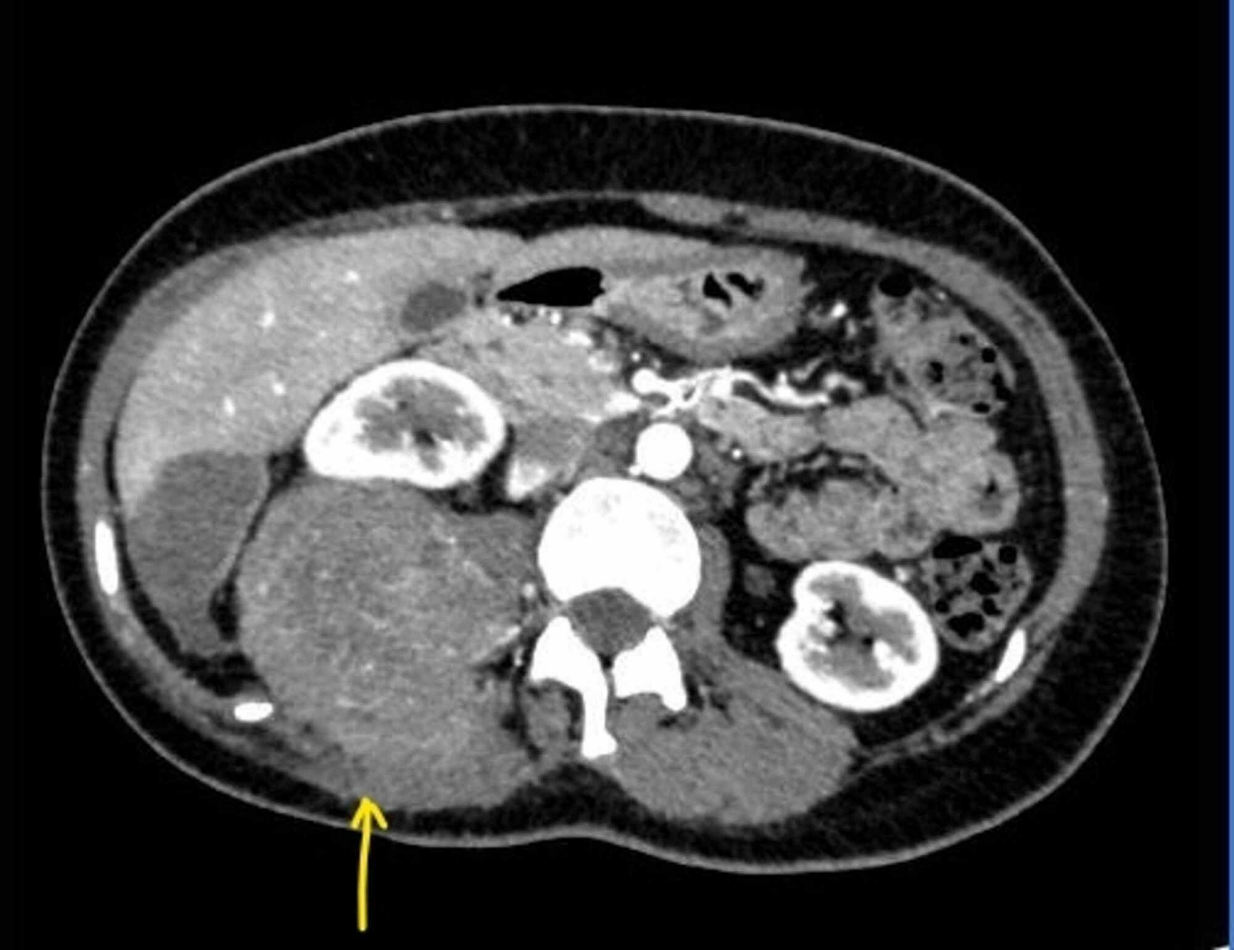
Contrast-enhanced CT image of tumor displacing right kidney.
